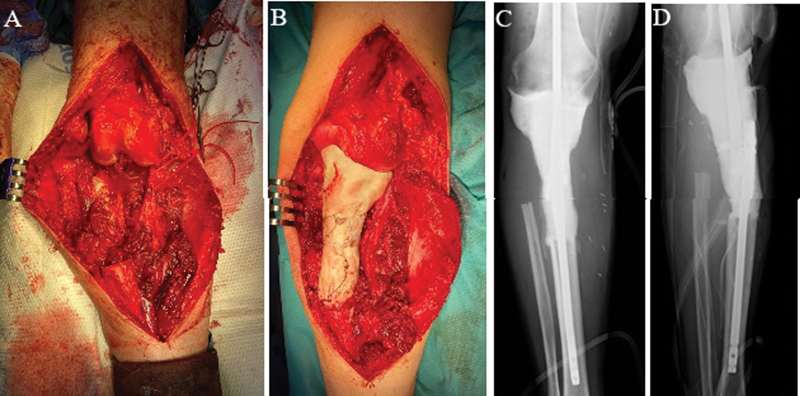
Images of the first surgical stage. A) The intraoperative photograph shows the tumour wide margin resection. The neurovascular bundle was identified, dissected and protected. A relevant dead space can be observed. B, C and D) The intraoperative photograph and the postoperative x-ray images show the temporary arthrodesis performed with two intramedullary nails (anterograde tibial and retrograde femoral). Antibiotic loaded cement was used to increase the construct stability, obliterate the dead space and locally deliver antibiotic.
